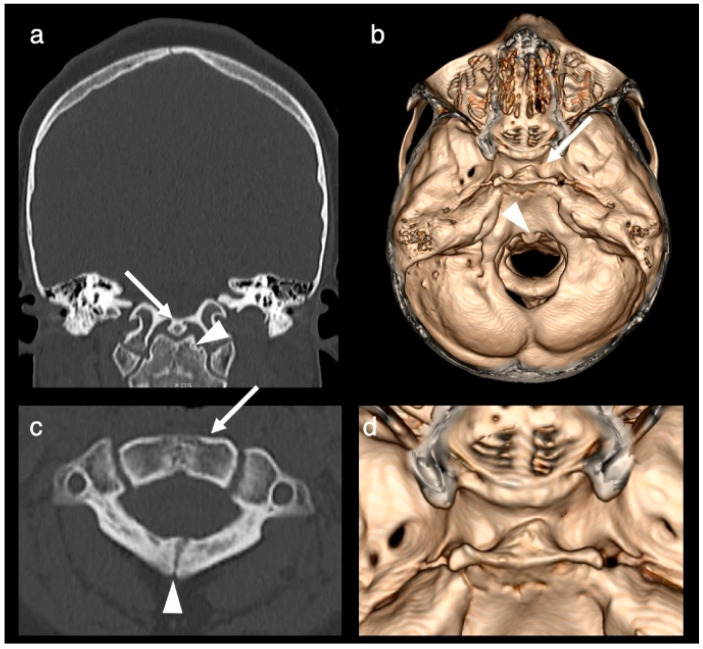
CT obtained at the age of 8 years. Coronal CT-image (bone algorithm; (a)), axial CT-3D reconstruction (b), axial CT-image (bone window, (c)) and axial CT-3D reconstruction (d). Images demonstrate a broadened/duplicated odontoid process (arrowhead in (a)), a bony fusion of a midline ossicle with the clivus (arrow in (a) and arrowhead in (b)), a broadened base of C2 (arrow in (c)), a congenital absence of the anterior arch of the atlas (c), an incomplete fusion of the posterior synchondrosis (arrowhead in (c)) and a broad and shallow sella turcica, which is divided into two fossae by a midline osseous ridge (arrowhead in (b) and close up in (d)).
